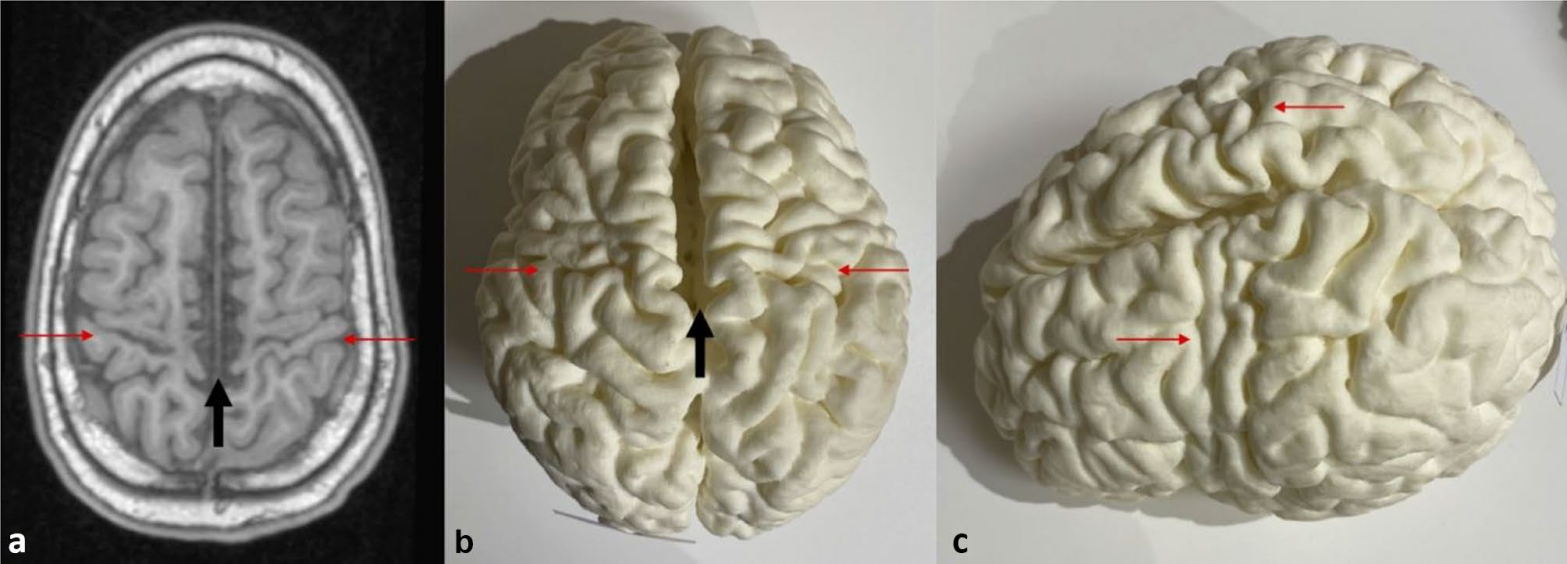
Comparison of axial T1W MRI image (a) with vertex view (b) and side oblique view (c) of the corresponding 3D printed model. The areas depicted by the red arrows correspond to subtle localized atrophy involving the peri-rolandic region of both cerebral hemispheres, sustained from a remote prior profound hypoxic ischemic injury in an 11-year 5-month-old girl. The black arrows depict the inter-hemispheric/lentiform separation due to the atrophy. The MRI image (a) depicts one aspect of the atrophy while the 3D printed model image (c) demonstrates the extension of involvement to the inferior and lateral aspects of the left pre-central gyrus to good effect
